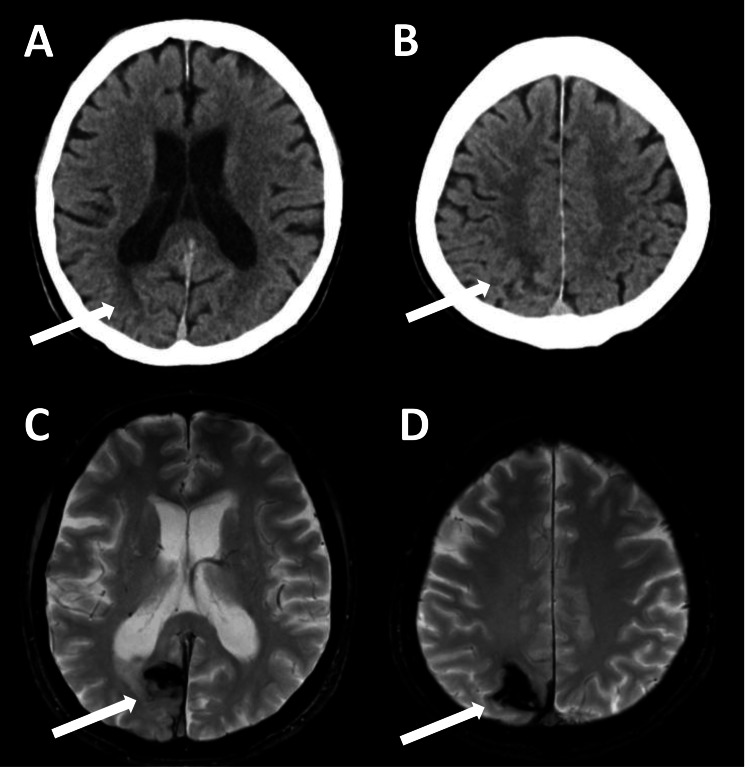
CT and MRI of the brain three months after the hemorrhagic event. CT (A, B) and T2*-weighted MRI (C, D) obtained three months after onset showing resolution of the hemorrhage and surrounding edema. The acute hematoma disappeared (A, B), and hemosiderin deposition was confined to the right precuneus (C, D).
